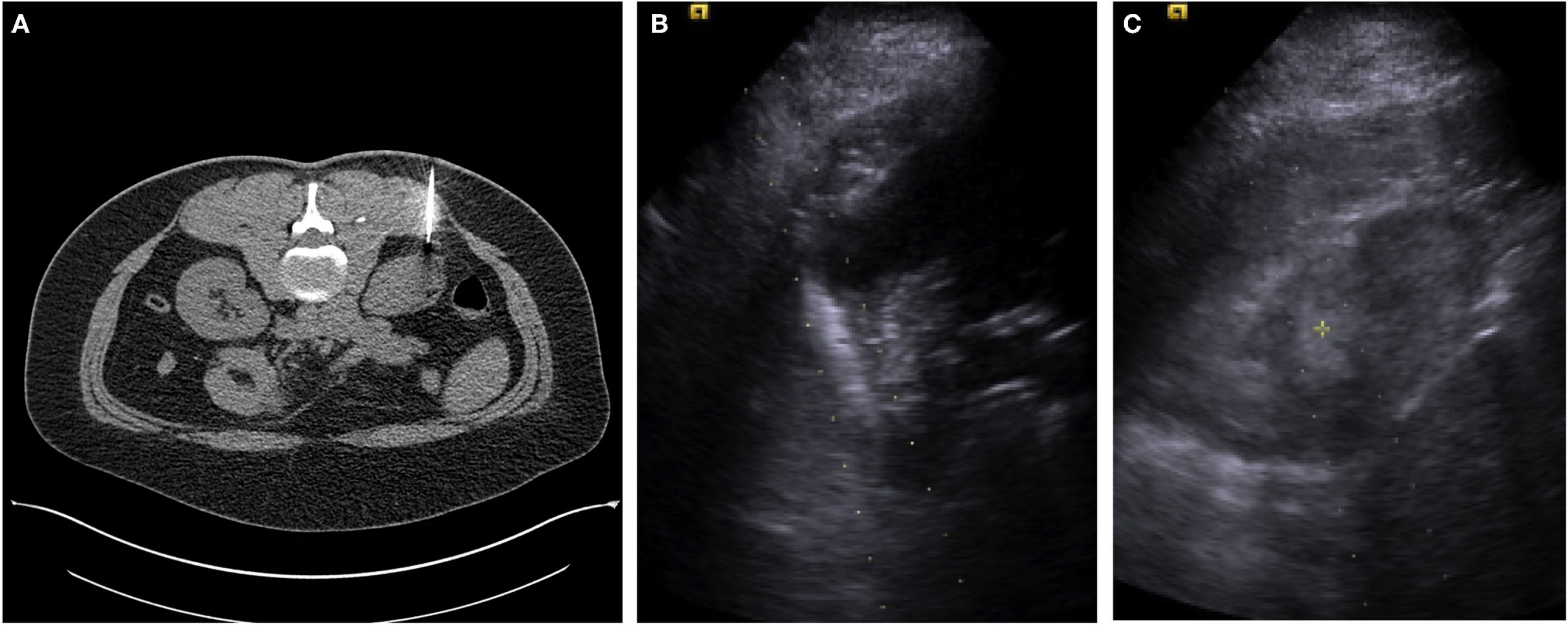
(A) A single axial CT image from a percutaneous CT guided biopsy using a coaxial technique and a 22-guage needle for fine needle aspirate sampling in a female with an incidentally detected 2.7 cm lower pole R renal mass. Pathology reported renal cell carcinoma, without subtyping. (B) An ultrasound image with the needle guide in place of a male with an incidentally detected 3.5 cm right upper pole renal mass. (C) A second ultrasound image from a percutaneous US guided biopsy using a coaxial technique and an 18-guage needle for core biopsy. Pathology reported as renal cell carcinoma, conventional clear cell type, Furman grade I–II.
